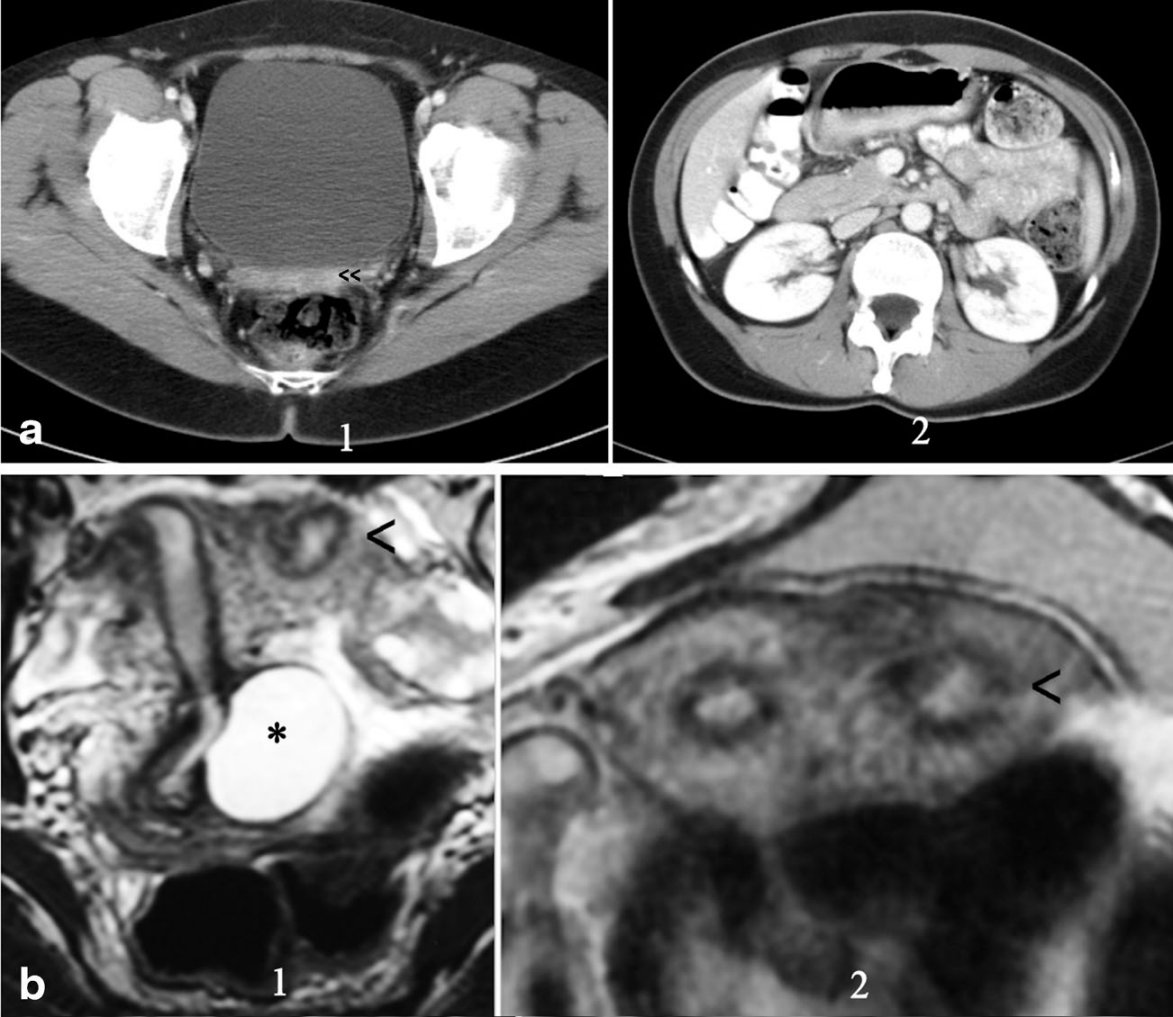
Rokitansky syndrome and unicornuate uterus. a (1) CT in a patient with Rokitansky syndrome showing the utero-vaginal rudimentary area under the bladder (<<). (2) Showing both normal kidneys. b Patient with a unicornuate uterus and cavitated rudimentary uterine horn. (1) Axial and (2) T2-weighted MR image (coronal cut) showing the left cavitated and rudimentary uterine horn (<). *Left retrocervical subperitoneal serous cyst corresponding to a Müllerian remnant (excised during laparoscopy). (Modified from Acién and Acién, Hum Reprod Update 2016;22:48–69, Fig. 3b, with permission.) The i.v. pyelography showed normal kidneys*Unicornuate uterus* comes in several variations, based on the degree of development and absence of communication to the contralateral side (Fig. 5b). It can be easily diagnosed with HSG, but attention must be given for the possibility of a didelphys uterus with unilateral canalisation and contrast injection. Nowadays, 3D-US is a better tool and MR is of special interest in the detection of a cavitated non-communicated uterine horn, which can also be observed with TVU. It must be remembered that in all isolated Müllerian anomalies both kidneys will be present.*Didelphys uterus* presents two completely detached hemiuteri (like two unicornuate uteri) with two cervices and a double vagina. The new ESHRE/ESGE classification system [22] has assimilated the didelphys to bicornuate uterus, including it as a complete bicorporeal uterus (U3/C2/V2). For the diagnostic imaging, the considerations made on the resorption of the septum and the bidirectional hypothesis of Müller et al. [26] have to be taken into account, and cases with a didelphic uterine corpus and simple (normal or septate) cervix and vagina can be found.*Bicornuate uterus* (Fig. 6a) includes complete (bicornis-bicollis uterus) and partial (bicornis-unicollis uterus) in the AFS/ASRM classification [19] or partial, complete and bicorporeal septate uterus in the new ESHRE/ESGE classification [22]. Some cases can have a cavitated non-communicating horn, and their inclusion as a bicornuate/septate or unicornuate uterus is discussed (see Fig. 5b). Currently, sonohysterography, 3D-US and specially the MR may provide the differential diagnosis with the septate uterus without the need of laparoscopy. In a pelvic MR, a significant fundal cleft (>1 cm) indicates no fusion of the upper-mid uterine horns [19, 46]. However, if this distance measures less than 1 cm, then a septate uterus would be present [46]. In the ESHRE/ESGE classification system [22], class U3 (bicorporeal uterus) is defined by an external indentation of >50 % of the uterine wall thickness, whereas in the complete bicorporeal uterus (U3b), the width of the fundal indentation at the midline is >150 % of the uterine wall thickness.
